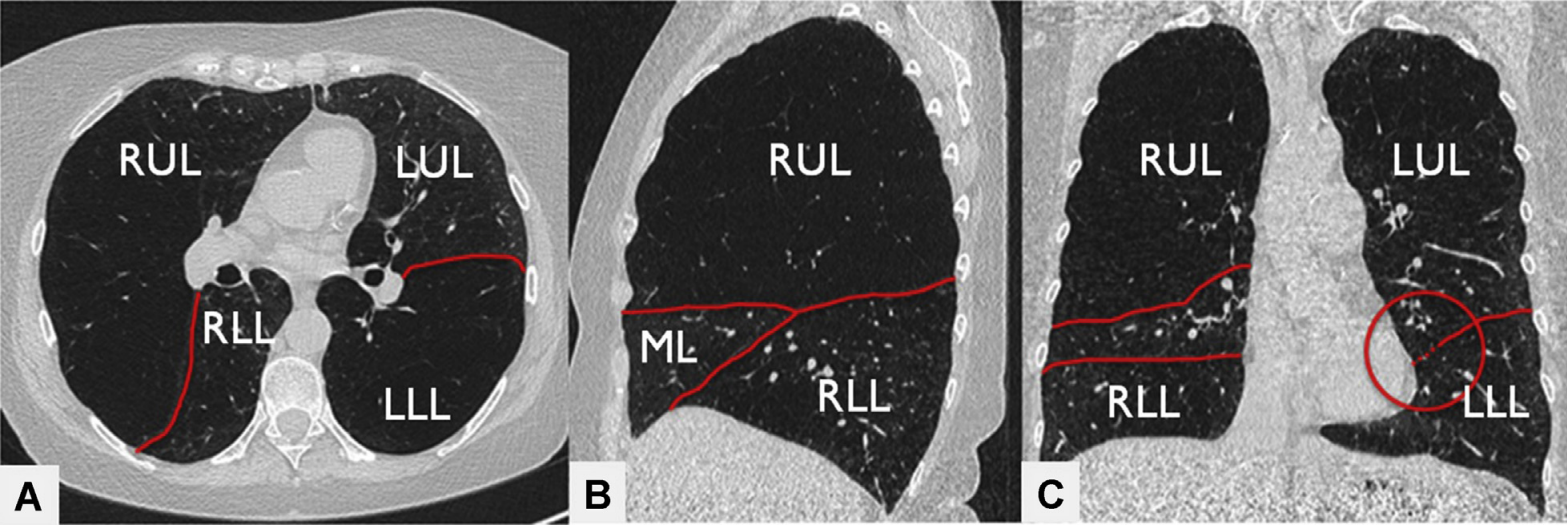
A-C, Example of visual assessment approach of the high-resolution CT scan. A, Axial view; B, sagittal view; C, coronal view of 1 mm high-resolution CT scan reconstructions with the use of a sharp kernel. The red lines indicate the fissures. The right upper lobe shows more destruction compared with the other lobes. The fissures in the right lung look intact on all three views. The left major fissure looks intact in the axial view; however, in the coronal view, a small gap is visible. Based on these slices, with the use of a visual assessment, the right upper lobe is a potential Zephyr endobronchial valve (Zephyr EBV; Pulmonx Corporation, Redwood City, CA) target lobe. LLL = left lower lobe; LUL = left upper lobe; ML = middle lobe; RLL = right lower lobe; RUL = right upper lobe.
